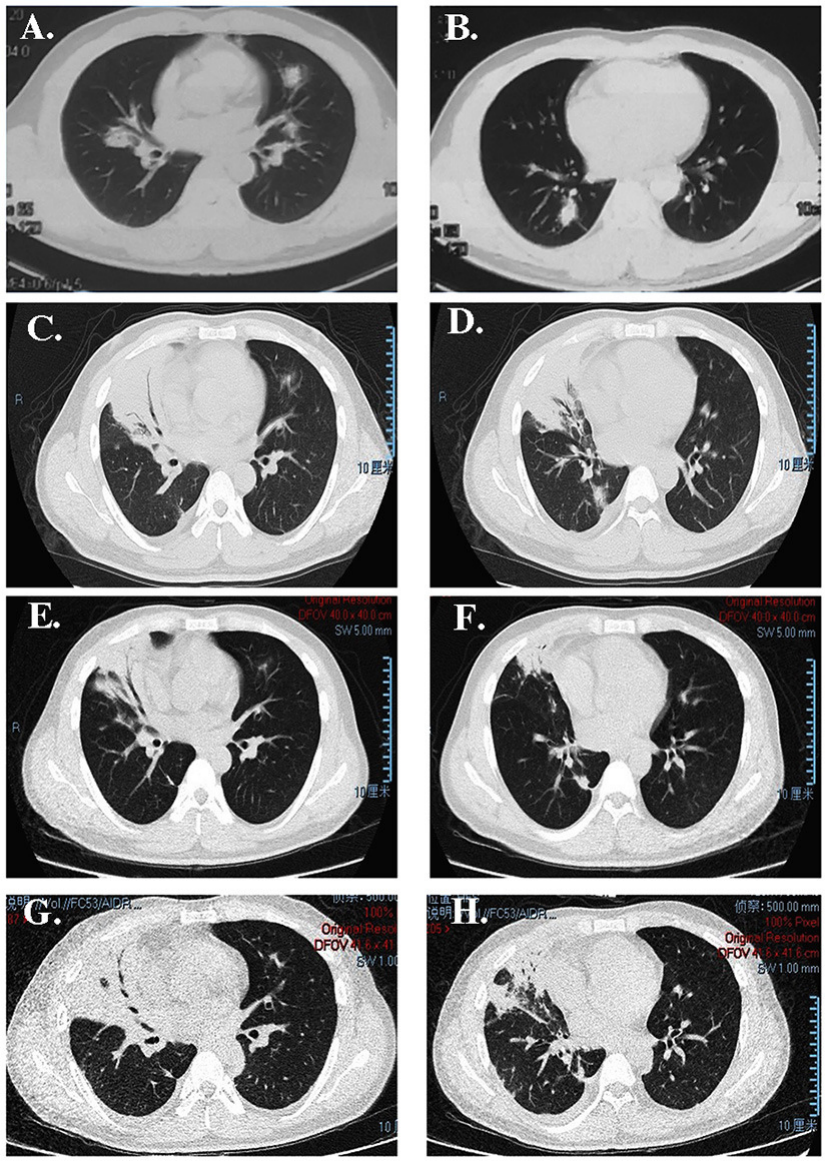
CT image showing (A, B) some nodules on the right upper lobe; (C, D) consolidation of the right upper lobe with an air bronchogram after antibiotic therapy administration in our hospital; (E, F) that the lesion was absorbed after treatment with dexamethasone; (G, H) more extensive lesions than previously observed after re-administering corticosteroids.
